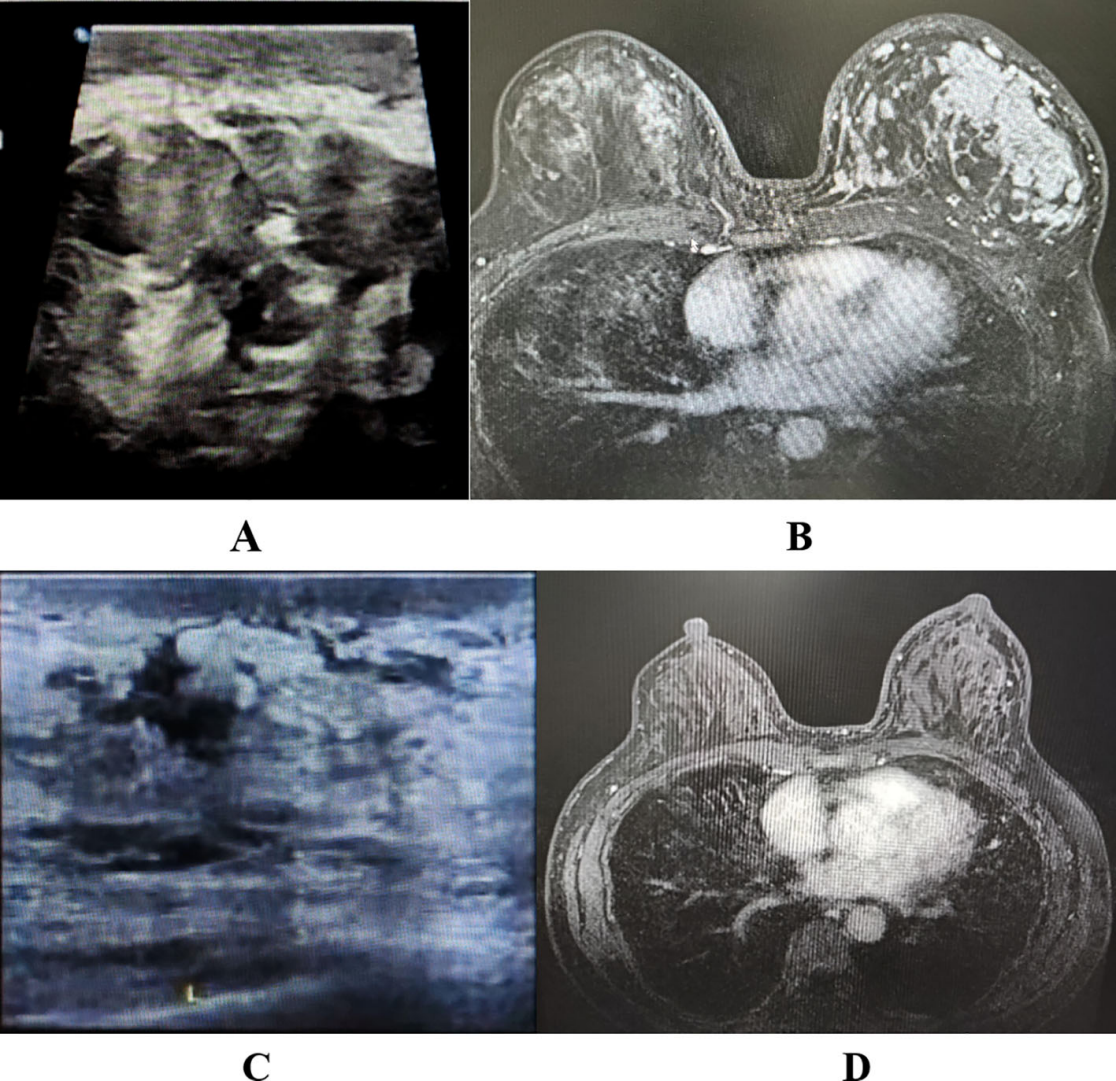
Pre-chemotherapy and post-chemotherapy diagnostic imaging of a patient with inflammatory breast cancer. Pre-treatment ultrasound (A) showing edema of the left breast skin and a hypoechoic area within the glandular tissue with indistinct borders and an irregular shape. Pre-treatment MRI (B) showing thickening of the left breast skin and multiple heterogeneously enhancing masses with poorly defined, coalescing margins within the glandular tissue, measuring approximately 112 × 45 mm in the largest cross-section. Post-treatment ultrasound (C) demonstrating persistent edema of the left breast skin and an irregular, patchy hypoechoic area within the glandular tissue with indistinct borders, showing a reduction in size compared to the pre-treatment scan. Post-treatment MRI (D) demonstrating mild skin thickening and multiple nodular signal foci with poorly defined, coalescing margins within the left breast, measuring approximately 35 × 10 mm in the largest cross-section.
